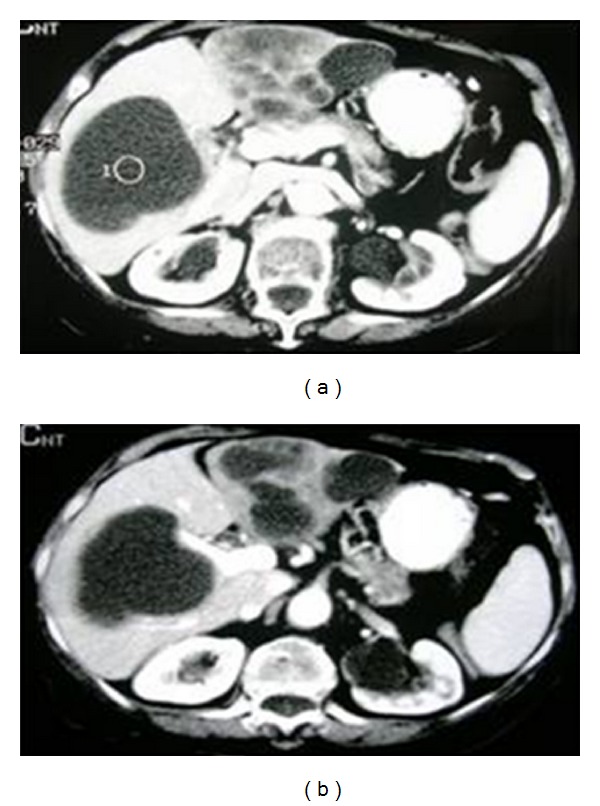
CT scan demonstrating cystic lesions or hypodense hepatic masses covering almost the entire part of the hepatic parenchyma.
